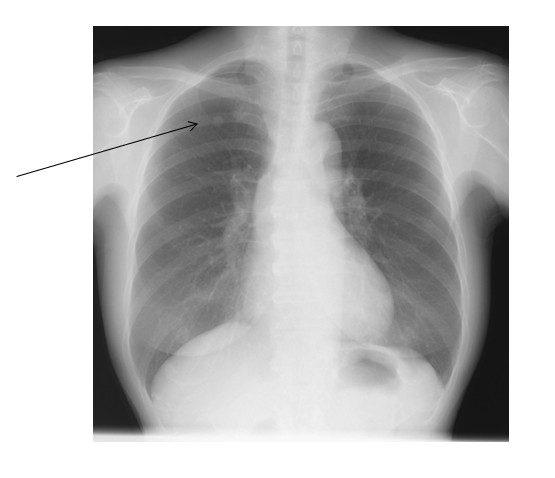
Chest radiography showing a nodular shadow in the upper lung field.
